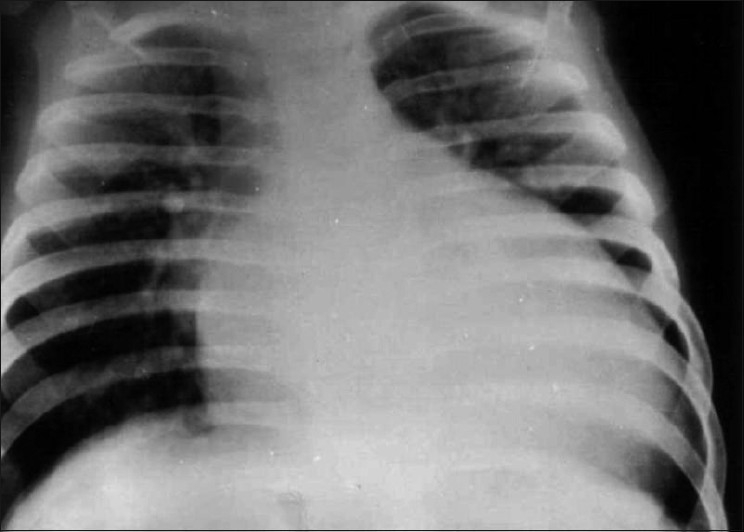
Radiographic finding of infant with ALCAPA shows cardiomegaly and pulmonary congestion
